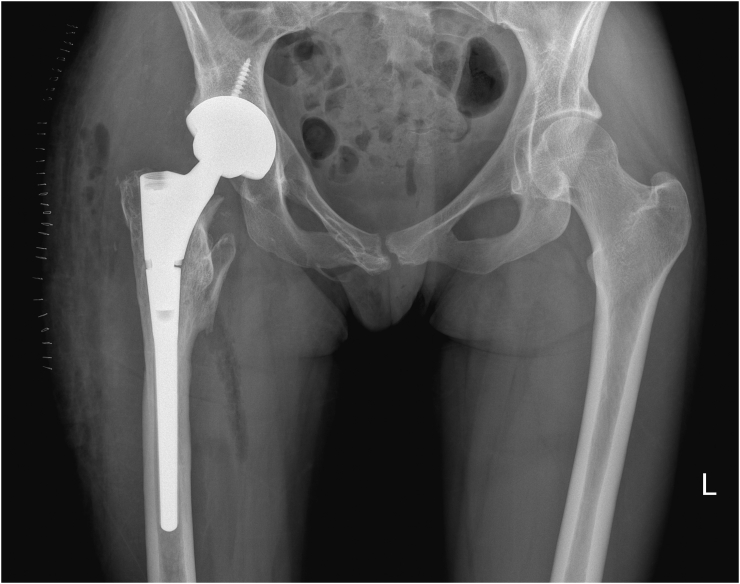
Standing AP pelvic radiograph taken after total hip arthroplasty.
